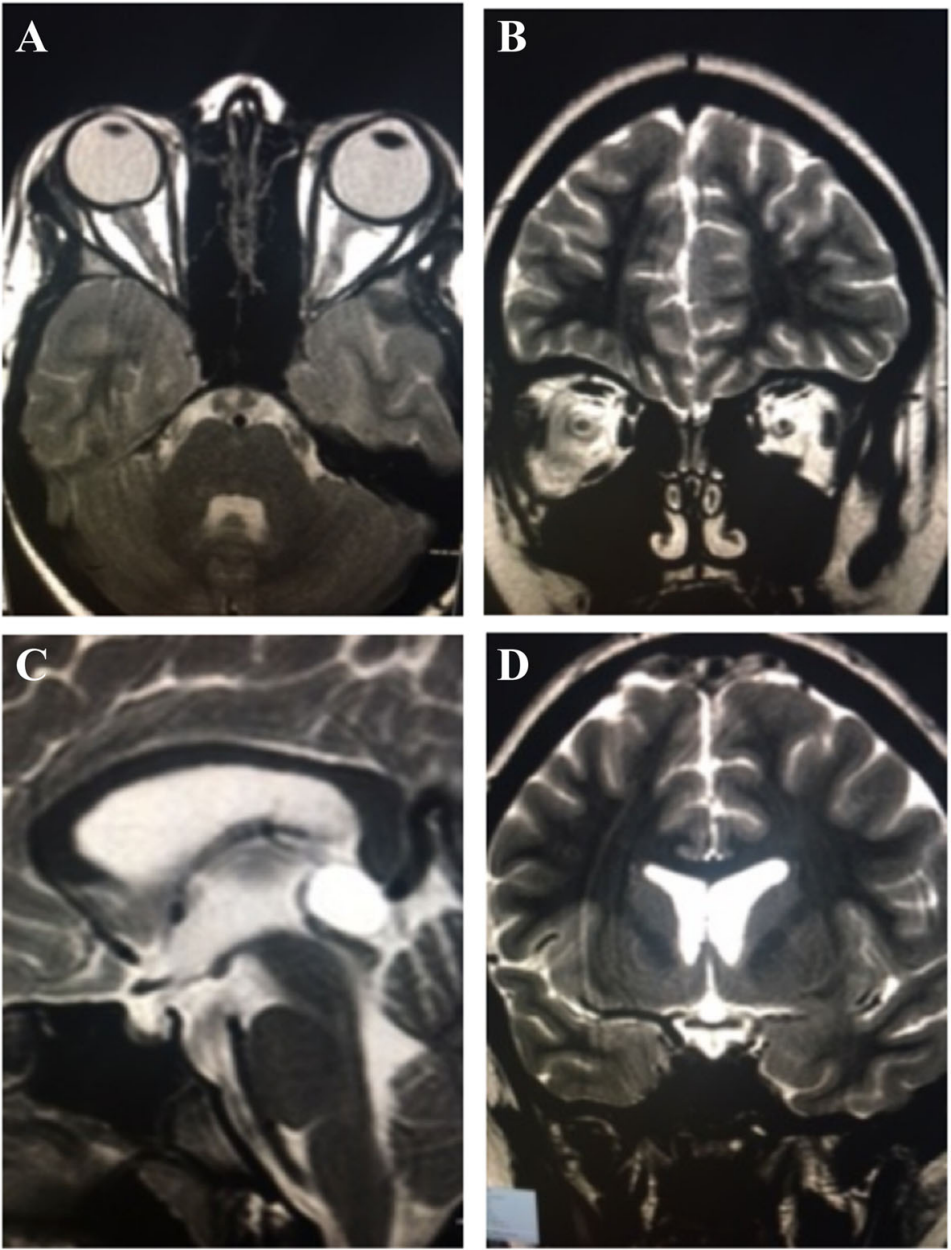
Brain Magnetic Resonance Imaging MRI TSE sequences of the patient showing mild inversion of the optic papilla on the optic disk on both sides, with prominent subaracnoid space around the optic nerves (a-b), and a partially empty sella turcica (c-d)
